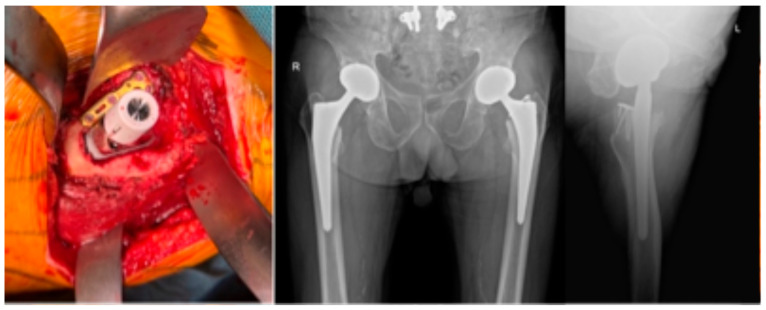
Intraoperative image showing a posterior intraoperative periprosthetic proximal femoral fracture at the base of the greater trochanter, fixed with a 37 mm L-Fusion Plate (DePuy Synthes, Warsaw, IN, USA) and four locking screws, along with its postoperative X-rays at 1 year.
